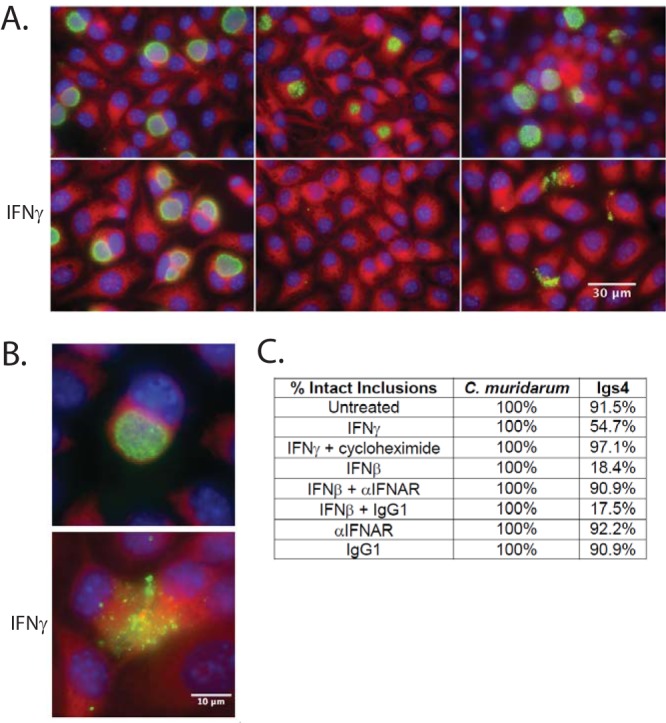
Type I and II interferons elicit lysis of Igs4 inclusions. (A) McCoy cell monolayers were infected with *C. muridarum* (CM), *C. trachomatis* (CT), or Igs4 at an MOI of 0.1 ± IFN-γ. The infected cells were fixed at 24 hpi and labeled with anti-LPS (green), DAPI (blue), and Evans blue (red). (B) Representative Igs4 inclusions labeled as described in panel A. (C) Mean percent intact inclusions in IFN-γ-, IFN-β-, α-IFNAR-, α-IFNAR plus IFN-β-, or CHX-treated McCoy cells at 24 hpi from two experiments performed in triplicate.
